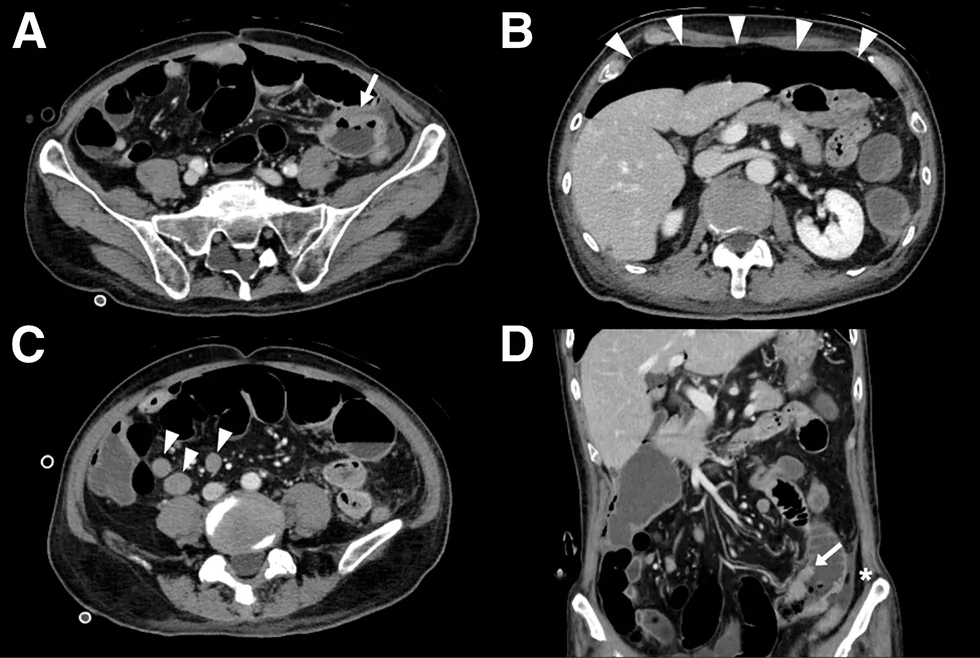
CT at Small-Bowel Perforation. (A) Axial image showing localized, contrast-enhancing wall thickening of the small bowel (arrow). (B) Axial image showing intraperitoneal free air (arrowheads). (C) Axial image showing enlarged mesenteric lymph nodes (arrowheads). (D) Coronal image showing the relationship between the small-bowel lesion (arrow) and ascites (asterisk).
